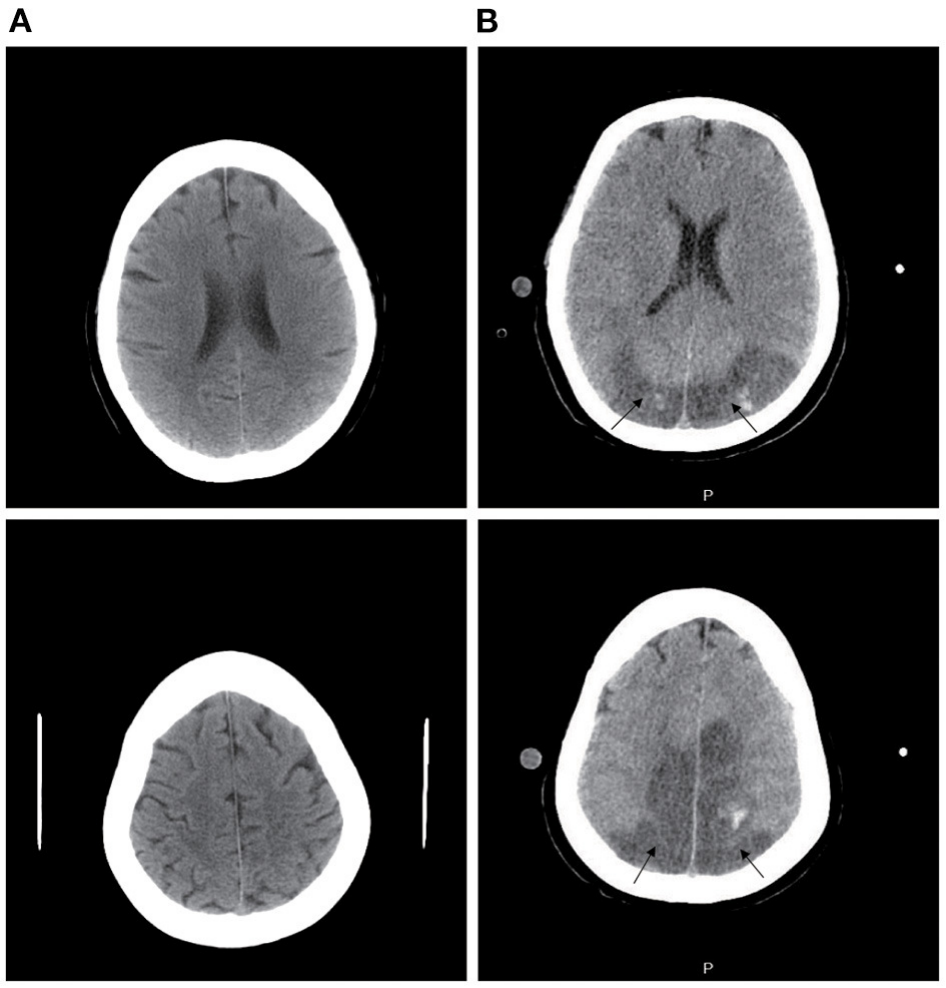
Computed tomography (CT) image of the head. (A) CT of the head is normal at the ECMO stage. (B) CT of the head shows bilateral parieto-occipital low-density lesions at day 3 after transplantation (arrowheads).
